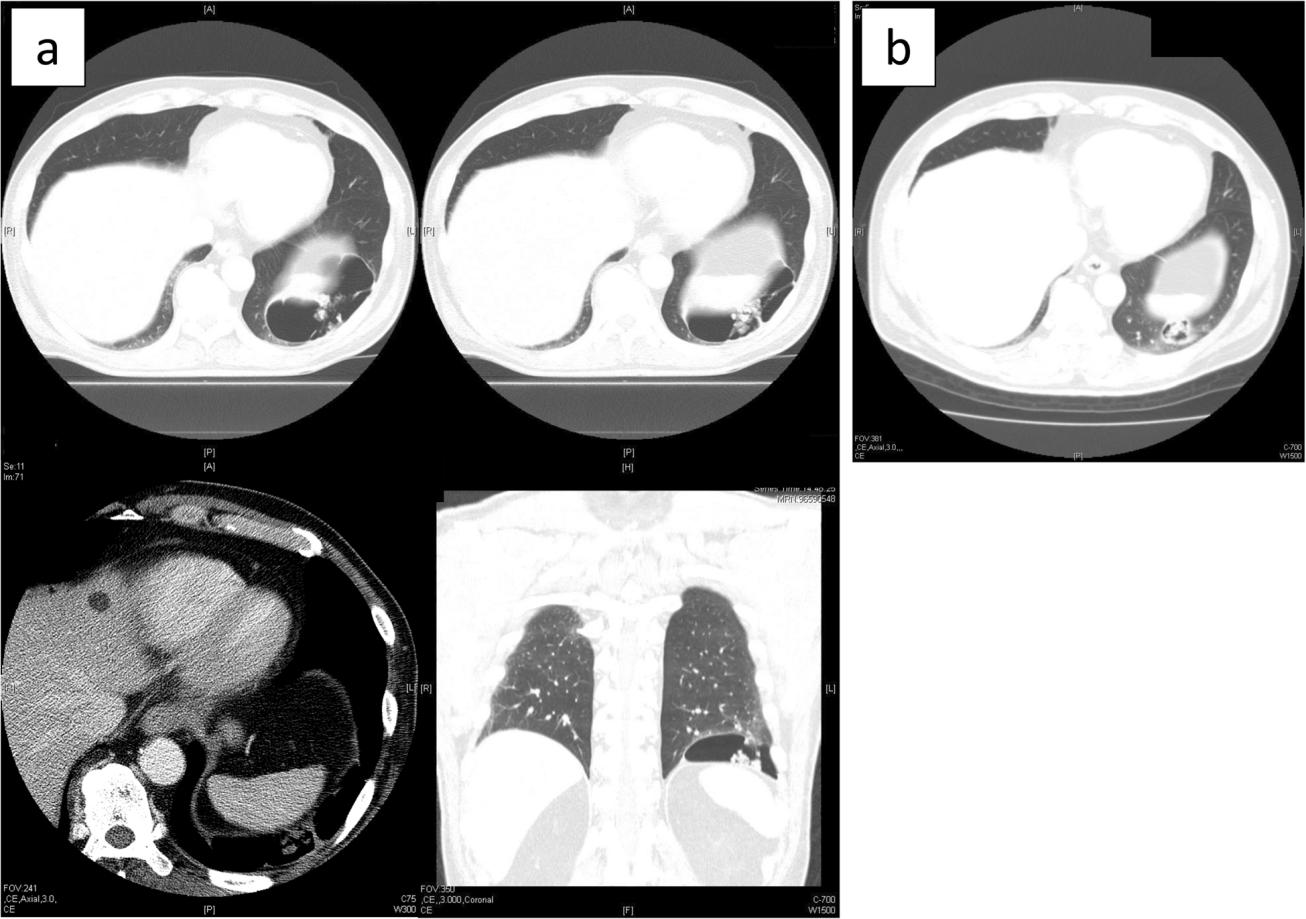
Chest CT showed a 9-cm cystic tumor in the left lower lobe, with small nodules aggregated in the cyst. The small nodules were slightly enhanced on contrast-enhanced CT (a). The cystic tumor had been found on abdominal CT 12 years previously (b)
